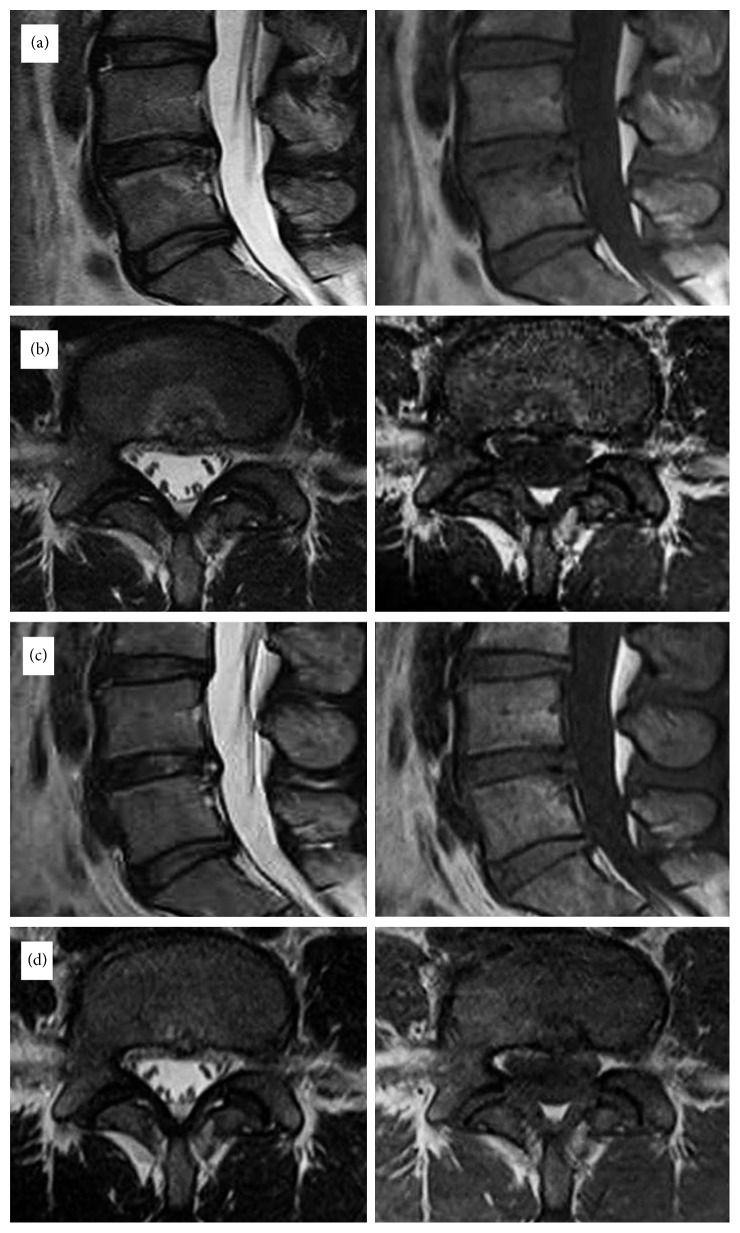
Magnetic resonance imaging (MRI) of a 31-year-old female patient who had suffered severe back pain and bilateral referred leg pain for more than 2 years. This patient's symptom improved significantly after TEBLA at the L4-L5 level. The preoperative VAS score of 8 decreased to 1 postoperatively. Preoperative T1WI and T2WI lumbar magnetic resonance imaging (MRI) sagittal (a) and axial (b) views with Modic type 1 changes. Postoperative T1WI and T2WI lumbar MRI sagittal (c) and axial (d) views showing resolution of the Modic reaction.
